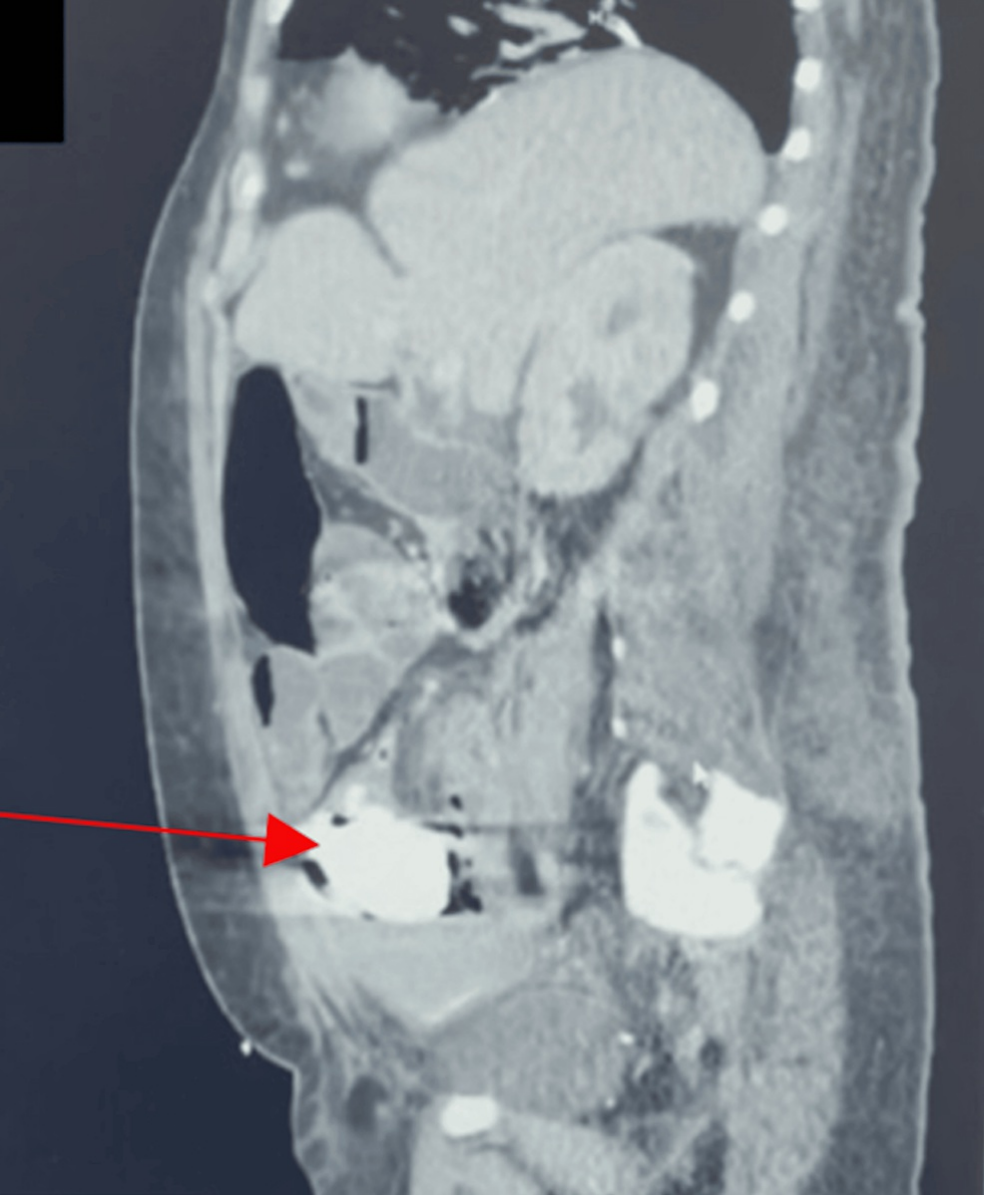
Sagittal CT of abdomen and pelvis with/without contrast showing barium impaction (red arrow) in sigmoid colon CT: Computed tomography
